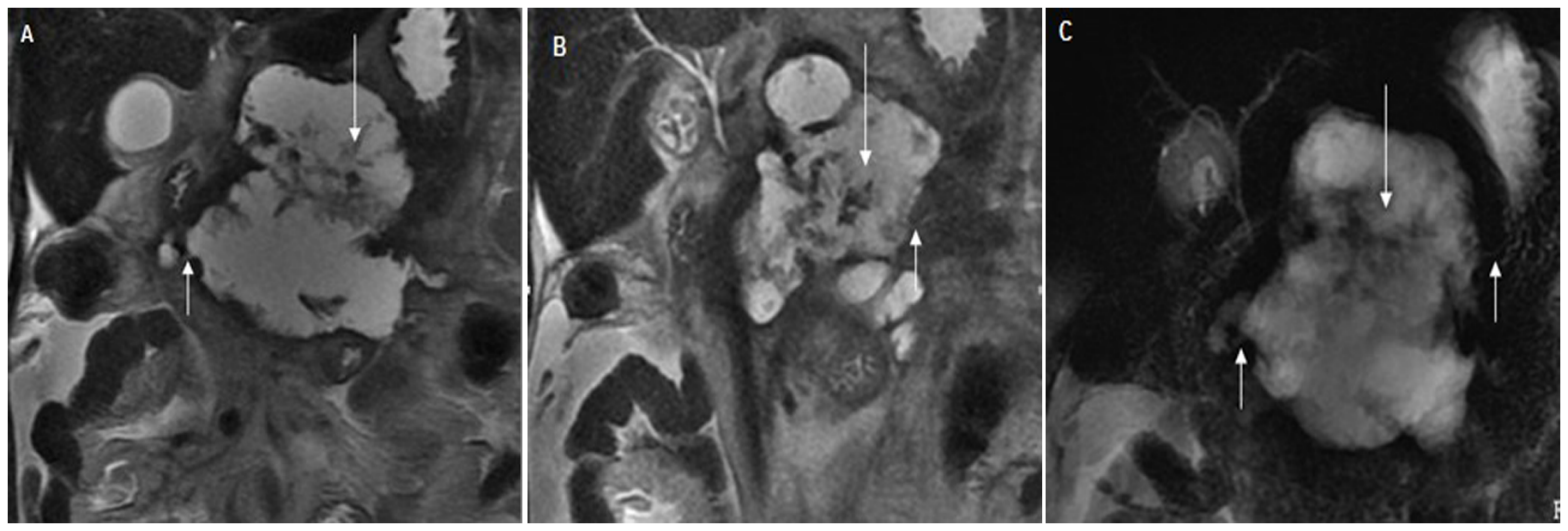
Pancreatic duct disruption in a 44-year-old female with acute pancreatitis. The Acute Physiology And Chronic Healthy Evaluation II score was 15, and the magnetic resonance severity index was 10. (A), (B) Coronal single-shot fast spin-echo T2-weighted images and (C) magnetic resonance cholangiopancreatography show a complex 8.5-cm-diameter ﬂuid collection (long arrow) in the head, body and proximal tail of the pancreas. The main pancreatic duct (short arrow) enters the fluid collection at an angle of approximately 90°.
